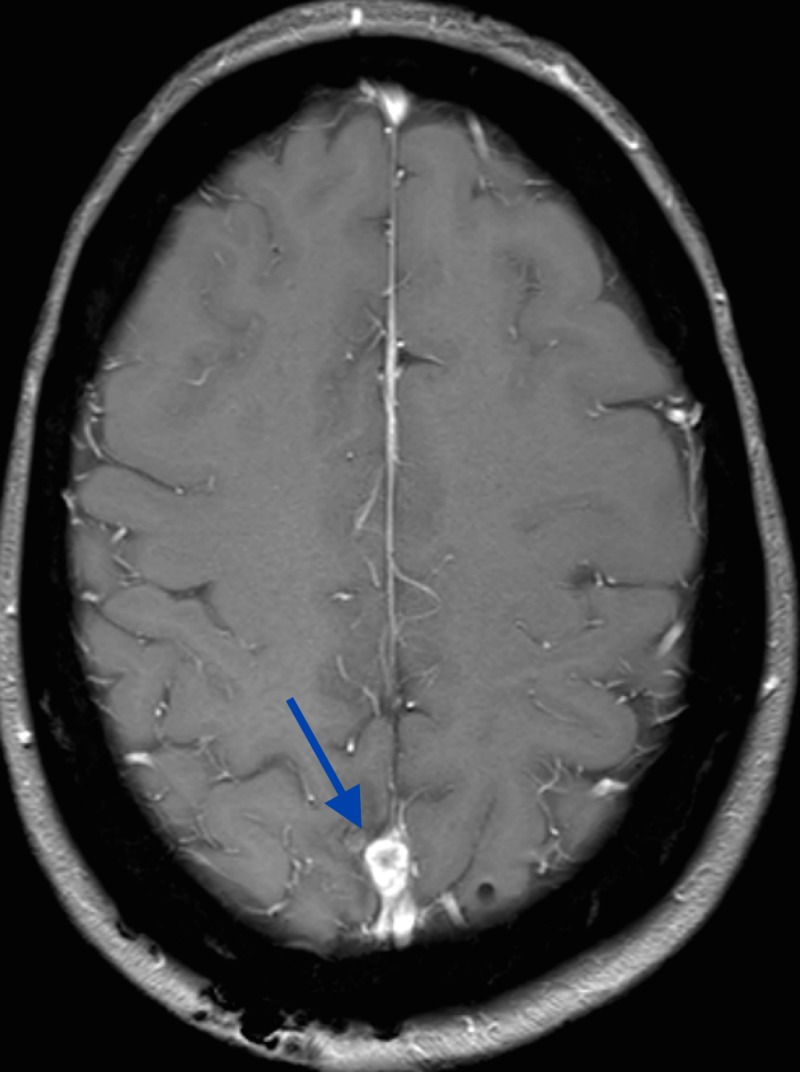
Axial T1 MRI demonstrates a recurrent right posterior falcine lesion measuring 11x7.5 mm MRI: magnetic resonance imaging
